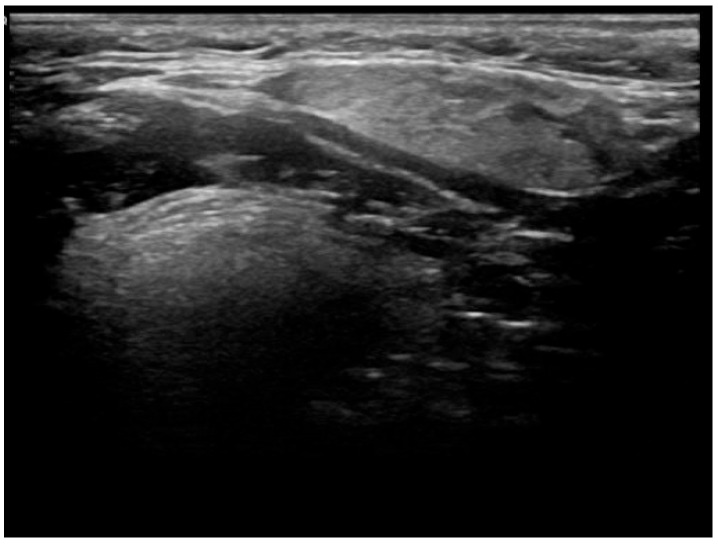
Patient X—Follow-up ultrasound scan of affected SMG. The previously identified abnormal area in the left SMG shows significant spontaneous resolution on follow-up imaging.
